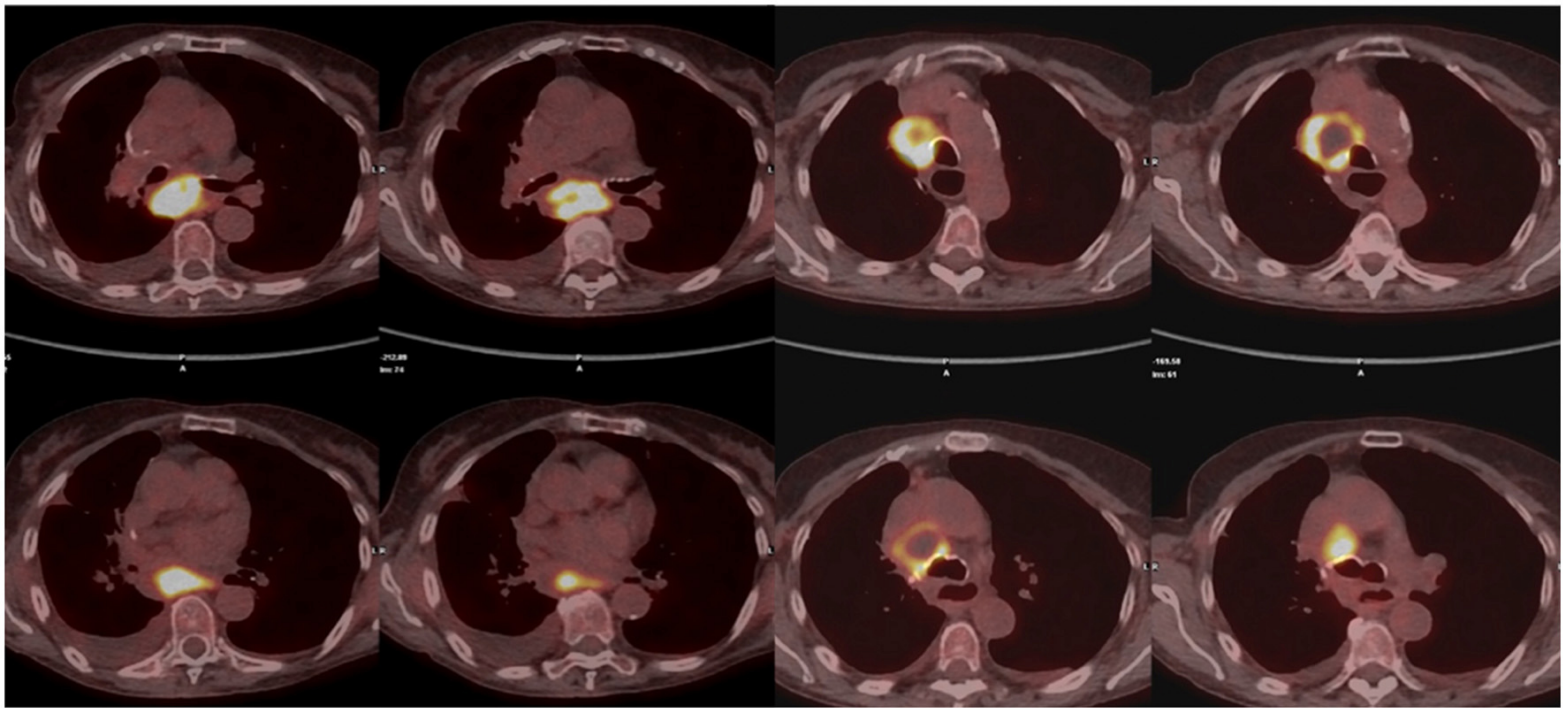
Subcarinal (7) and right inferior paratracheal (4R) FDG-PET positive lymph node.
